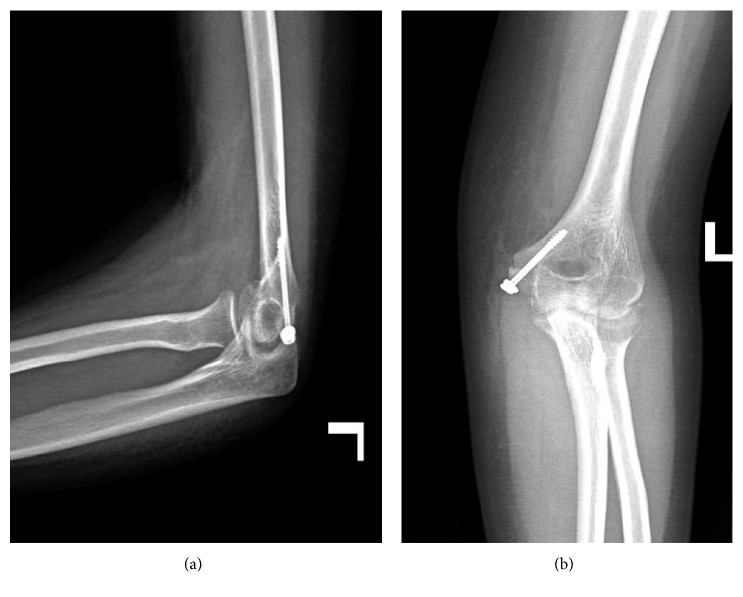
Left elbow control X-rays done at 3 weeks postop that showed proper integrity of the reduction.
